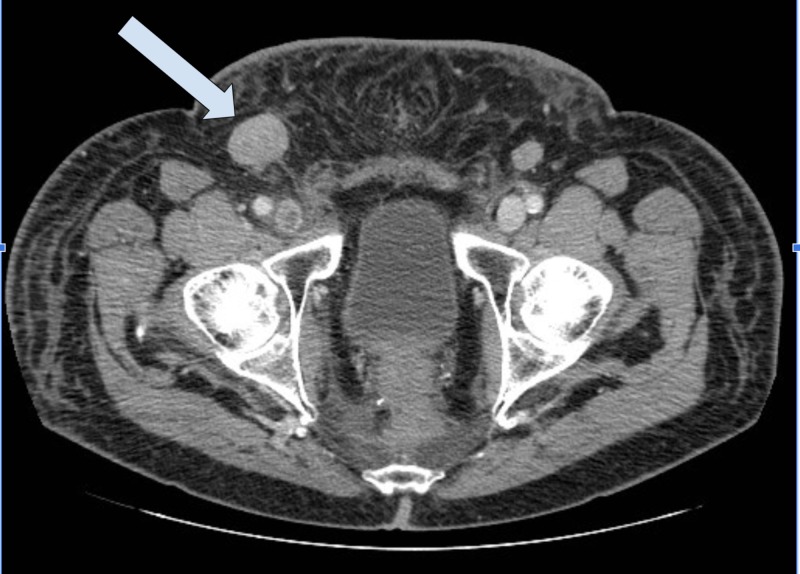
CT Abdomen and Pelvis with IV Contrast Interval worsening of inguinal lymphadenopathy, representing metastatic rectal cancer.
